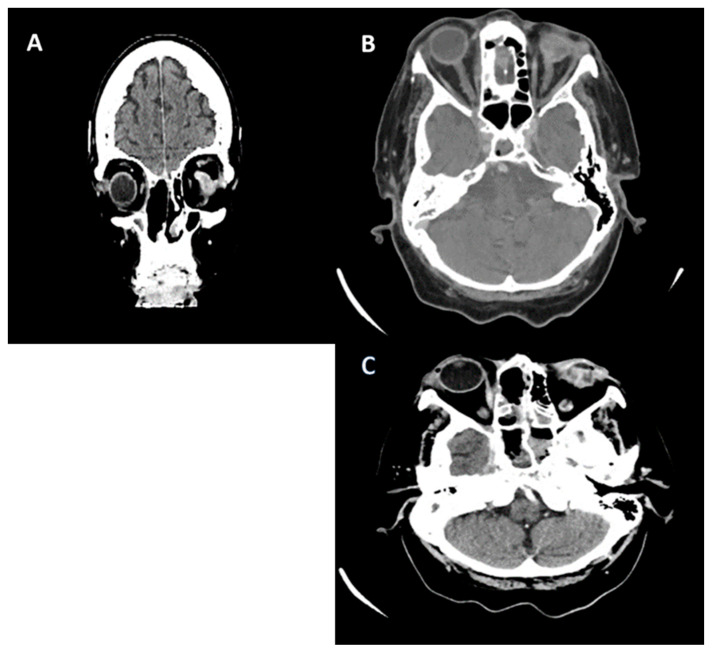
Cranial CT. (A) (scale bar equals 1.25 mm). Left ocular globe with loss of sphericity, reduced dimensions, and heterogeneous hyperdense appearance of the vitreous body with subcorneal and subscleral air infiltrates. (B,C) Thickening of the mucosa of the right maxillary sinus with lysis of the postero-superior wall, as well as the posterior right ethmoid with a collection showing fluid and parafluid densities at that level, with an iodophilic wall communicating with another collection with the same characteristics in the projection of the right sphenoid, which presents lysis of the anterior wall, lysis of the anterior ethmoid cells, and the nasal bones on the right side, as well as a collection in the right frontal sinus.
